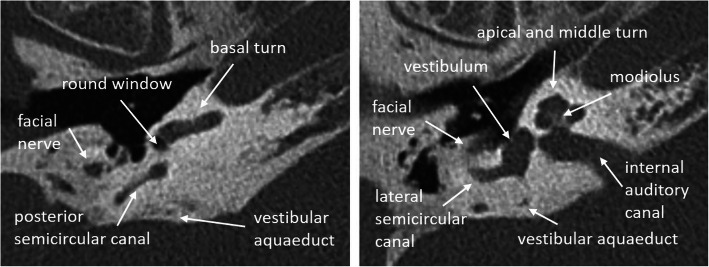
HRCT axial images of normal cochlear anatomy in a 74-year-old man
